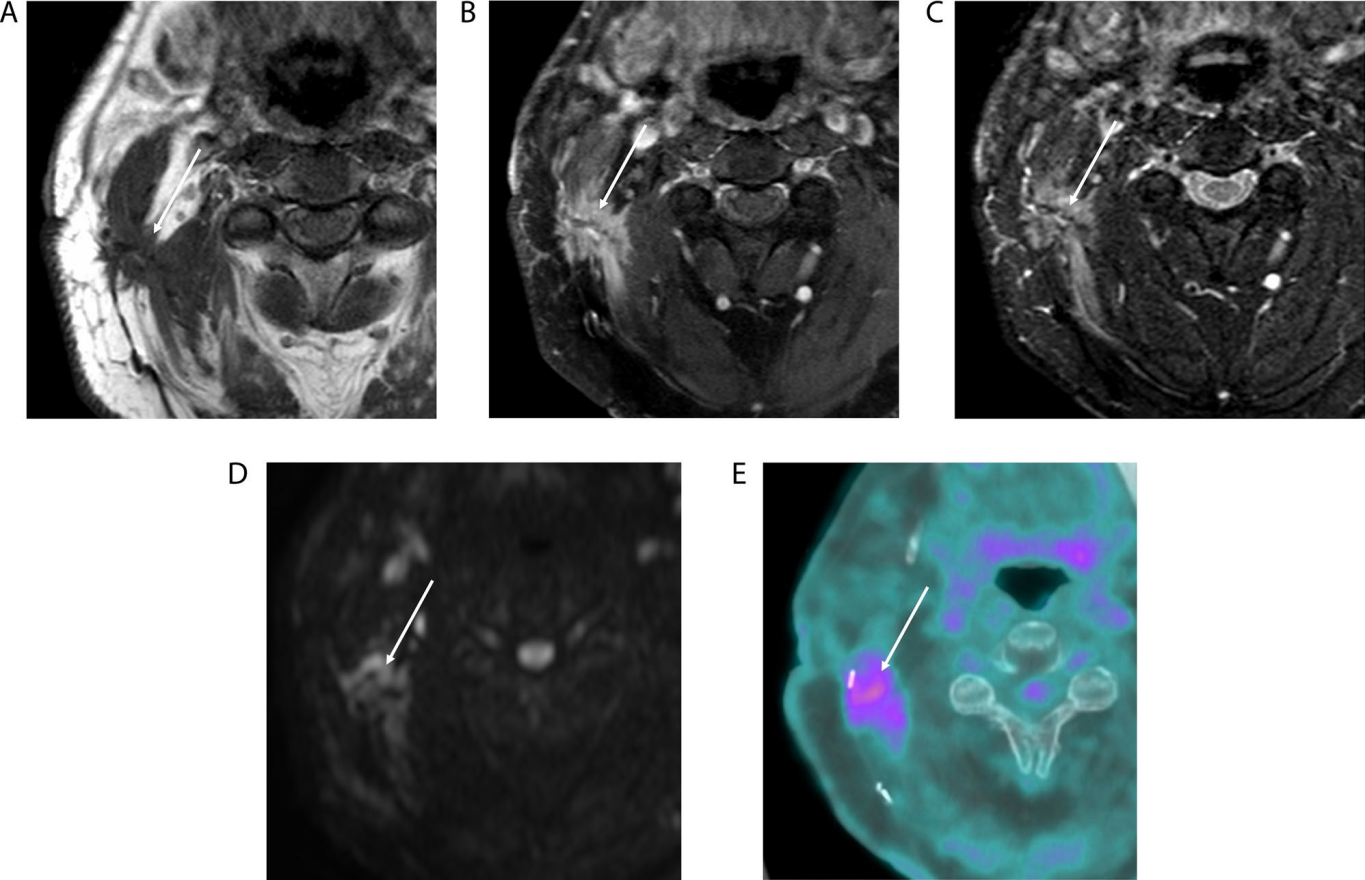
Recurrent HNSCCA at the deep margin of a free flap reconstruction, NI-RADS MRI primary category 3. Axial T1-weighted (A), Axial T1-weighted fat-suppressed contrast-enhanced(B), Axial T2-weighted fat-suppressed (C), and Axial diffusion-weighted MR (D) images demonstrate the utility of T2-weighted and diffusion-weighted images to distinguish recurrent tumor (arrows) from surrounding post treatment changes, with intermediate T2 signal intensity and low diffusivity, respectively. This is confirmed by axial fused FDG PET/CT (E) with corresponding intense FDG uptake
